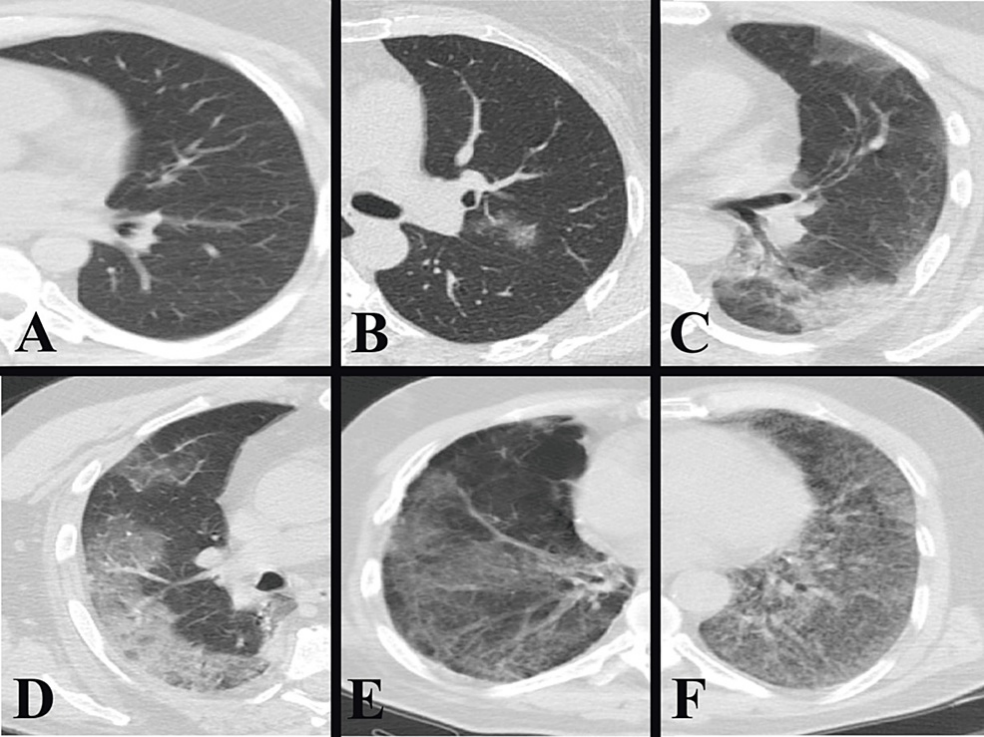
Thorax CT parenchyma scoring. Absence of parenchymal involvement score 0 (A), parenchymal involvement 0-5% score 1 (B), 5-25% score 2 (C), 25-50% score 3 (D), 50-75% score 4 (E), and 75-100% score 5 (F).
